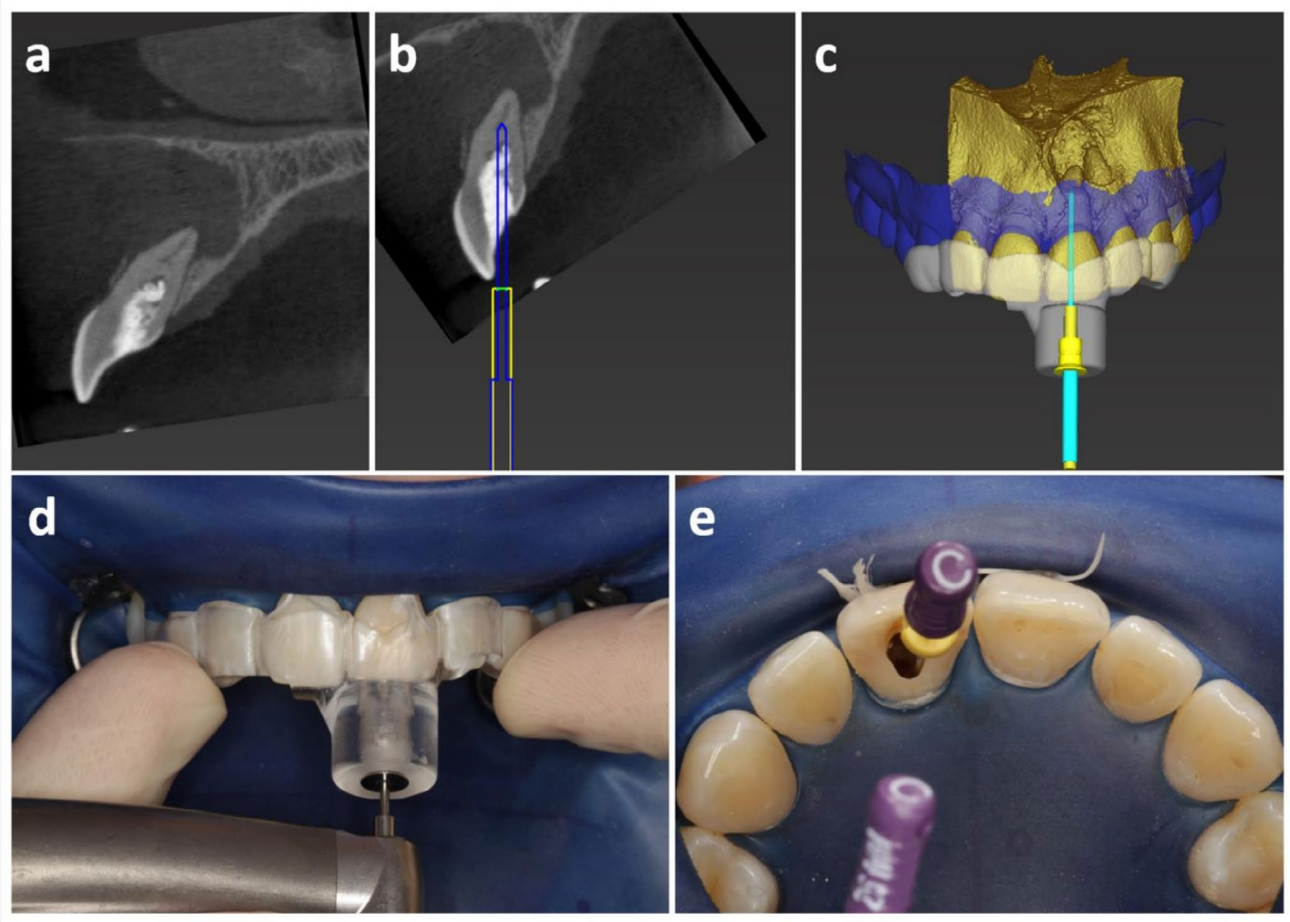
Case presentation of a template‐guided endodontic access cavity preparation (adapted from Connert et al. [34]). (a) Preoperative CBCT showing an unsuccessful root canal localization attempt by the referring dentist in a tooth severely affected by pulp canal calcification. (b) Virtual planning of the access cavity to the orifice of the calcified root canal. (c) Three‐dimensional representation of the virtual planning including the template. (d) Clinical execution of the guided access cavity preparation under rubber dam isolation. (e) Successful localization of the root canal.
